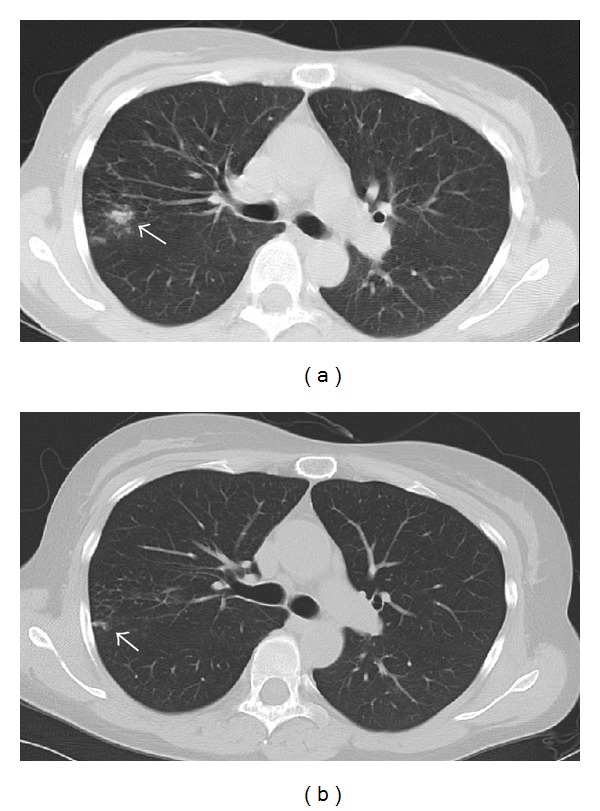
(a) Chest CT scan before starting posaconazole showing reticulonodular opacities in the right upper lobe. (b) CT scan one month after starting posaconazole showing resolution of most of the opacities.
